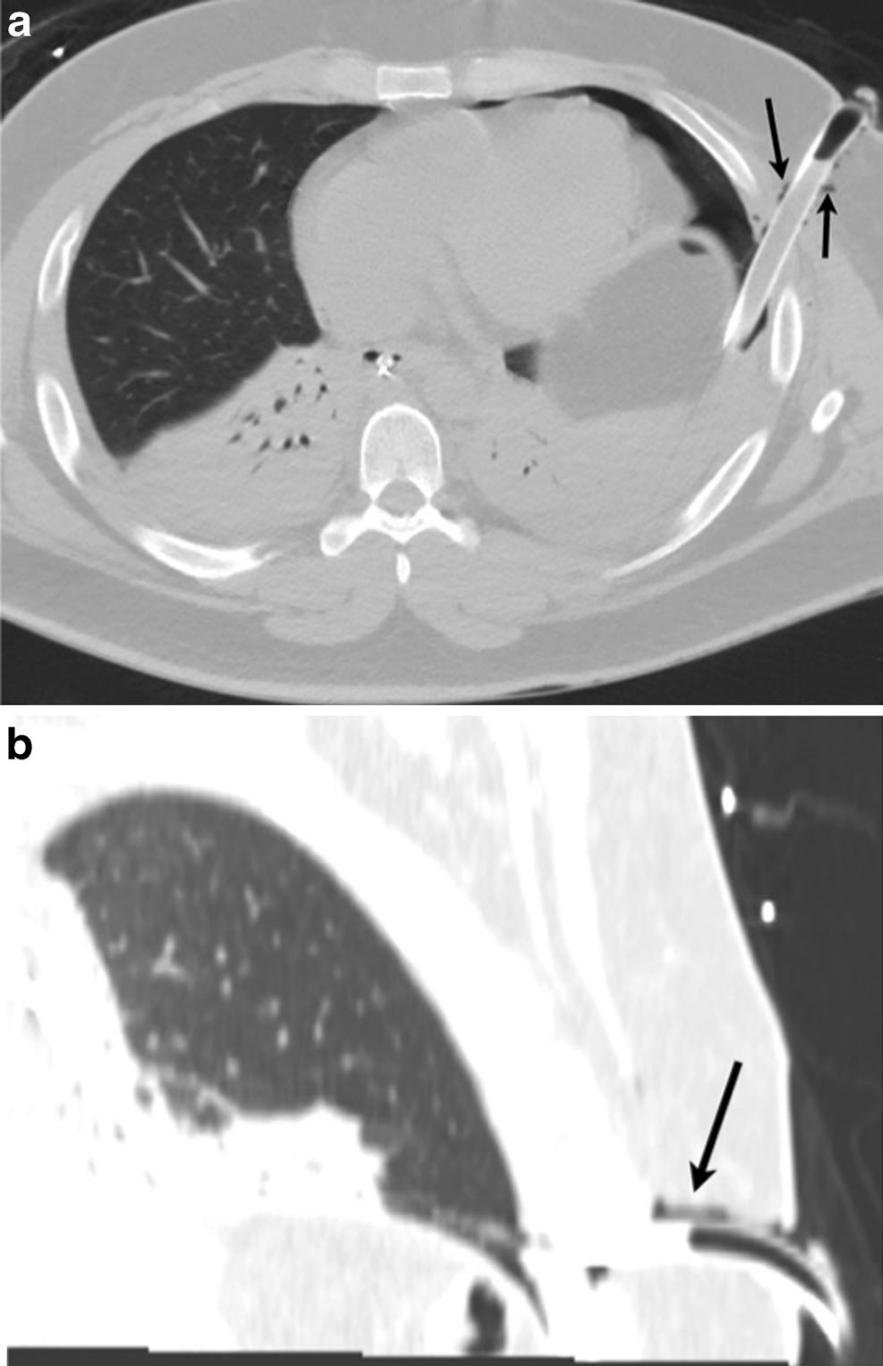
A 54-year- old-male status post aortic aneurysm repair presented with a persistent left pneumothorax. On axial (a) and coronal (b) CT images, air surrounds the portion of the chest tube coursing through the chest wall (*arrows*). This indicates an incomplete seal. If the site of thoracotomy is not optimally occluded with surgical dressing, or if the incision is too large relative to the tube, an air leak may develop. This leak allows air back into the pleural space during inspiration and results in a nonresolving pneumothorax
